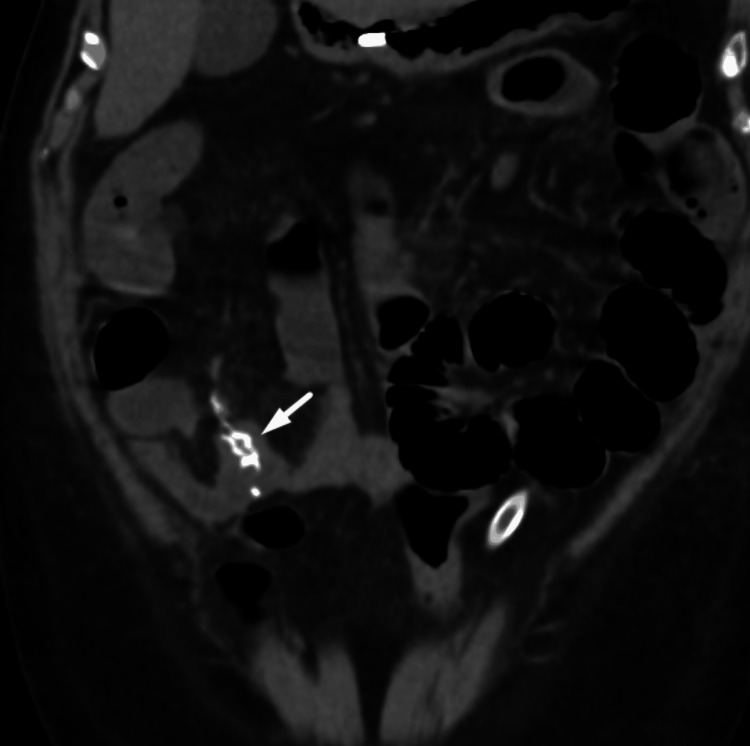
Abdominal CT scan image in coronal view showing anastamosis of the jejunum following resection of the jejunal growth (white arrow). CT: computed tomography
